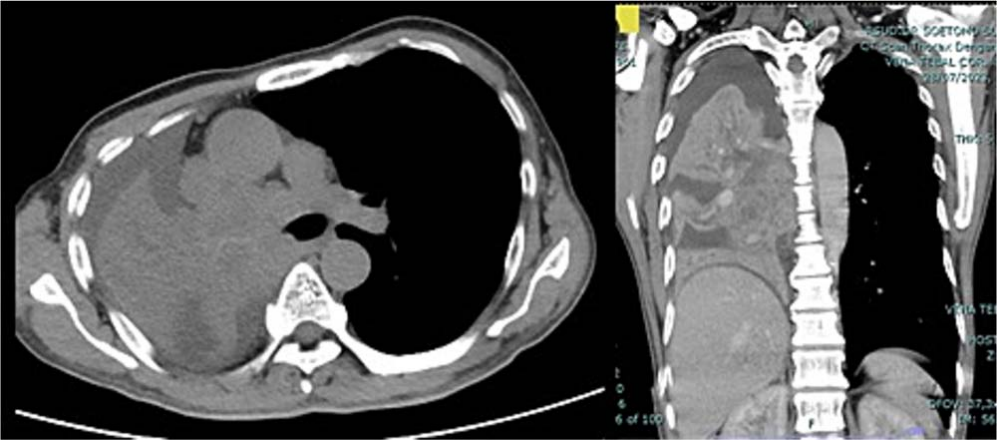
CT scan thorax with contrast showed enhancing solid mass measuring ±9.6 × 9.5 × 14.5 cm in the centre of the right lung extending to the entire right lung, middle, and subcarinal mediastinum mass.
